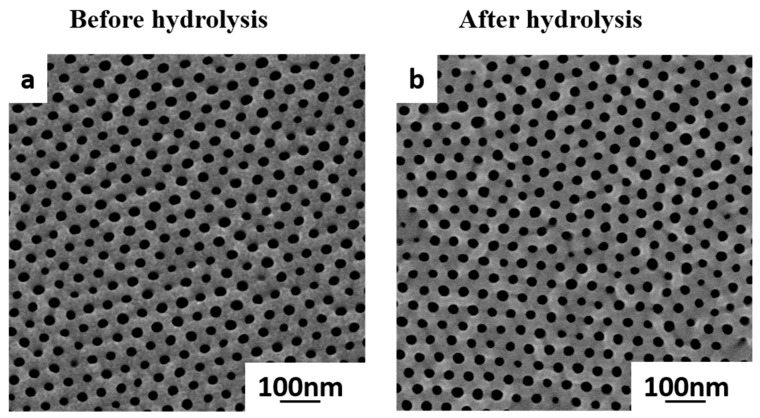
(a) SEM images of pristine PS_71_-*b*-P4VP_26_-*b*-PSMA_3_^145^ membrane before acidic hydrolysis and (b) PS_71_-*b*-P4VP_26_-*b*-PGMA_3_^145^ membrane.
